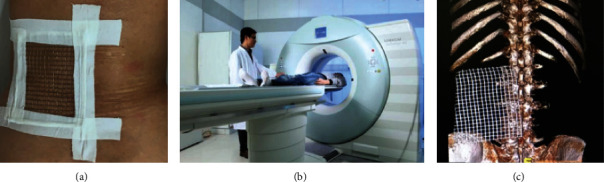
(a) Before the procedure, the body surface locator was put on the fracture vertebral body's body surface skin and secured appropriately. (b) Scanning the CT picture of the fractured vertebral body with the body surface locator. (c) A lumbar spine CT three-dimensional reconstruction picture using a body surface locator.
